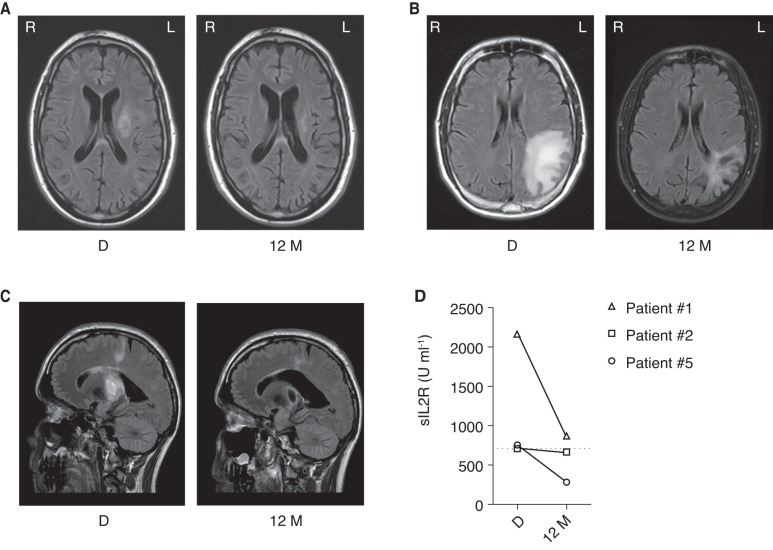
Regression of MRI lesions and serum sIL2R with infliximab therapy. MRI T_2_-weighted scans of (A) Patient #1, (B) Patient #2 and (C) Patient #3 at the time of diagnosis (D) and after 12 months of treatment with infliximab (12 M) is shown. (D) Serum sIL2 receptor (sIL2R) measurements after 12 months of treatment with infliximab (12 M) in comparison to time of diagnosis (D) in three patients with sarcoidosis and PML [*T* (2) = 1.65, *P* = 0.240, n.s.]. Grid line showing the upper limit of the reference range at <710 U ml^−1^. Statistical analysis was performed by paired two-tailed Student’s *t*-test.
